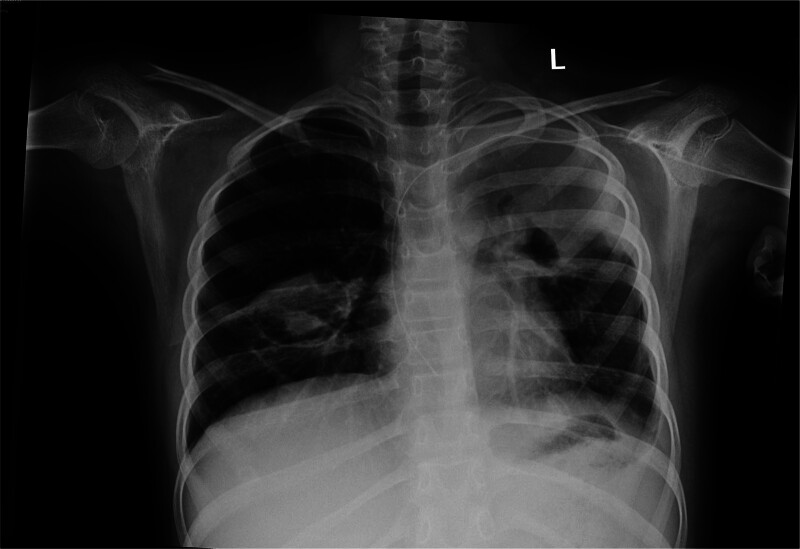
Chest radiography showed that both lungs had high-density flaky shadows, and the right lung had cavities at the lesion site.
